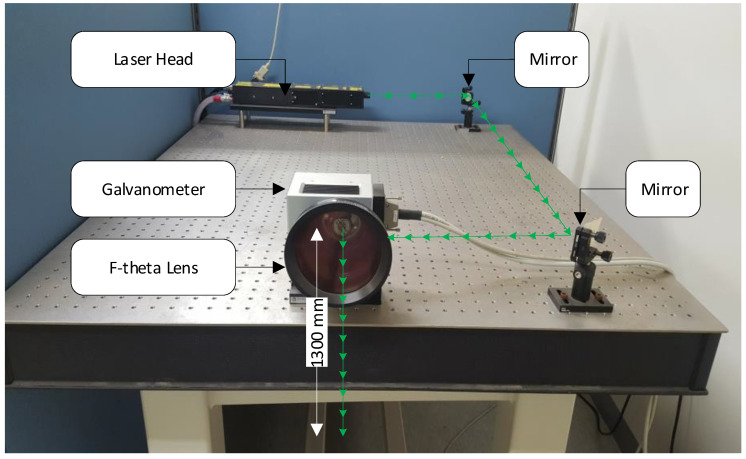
Experimental setup: A non-contact laser ultrasonic scanning system composed of a Q-switched Nd:YAG pulsed laser with a galvanometer for ultrasonic excitation scanning.
